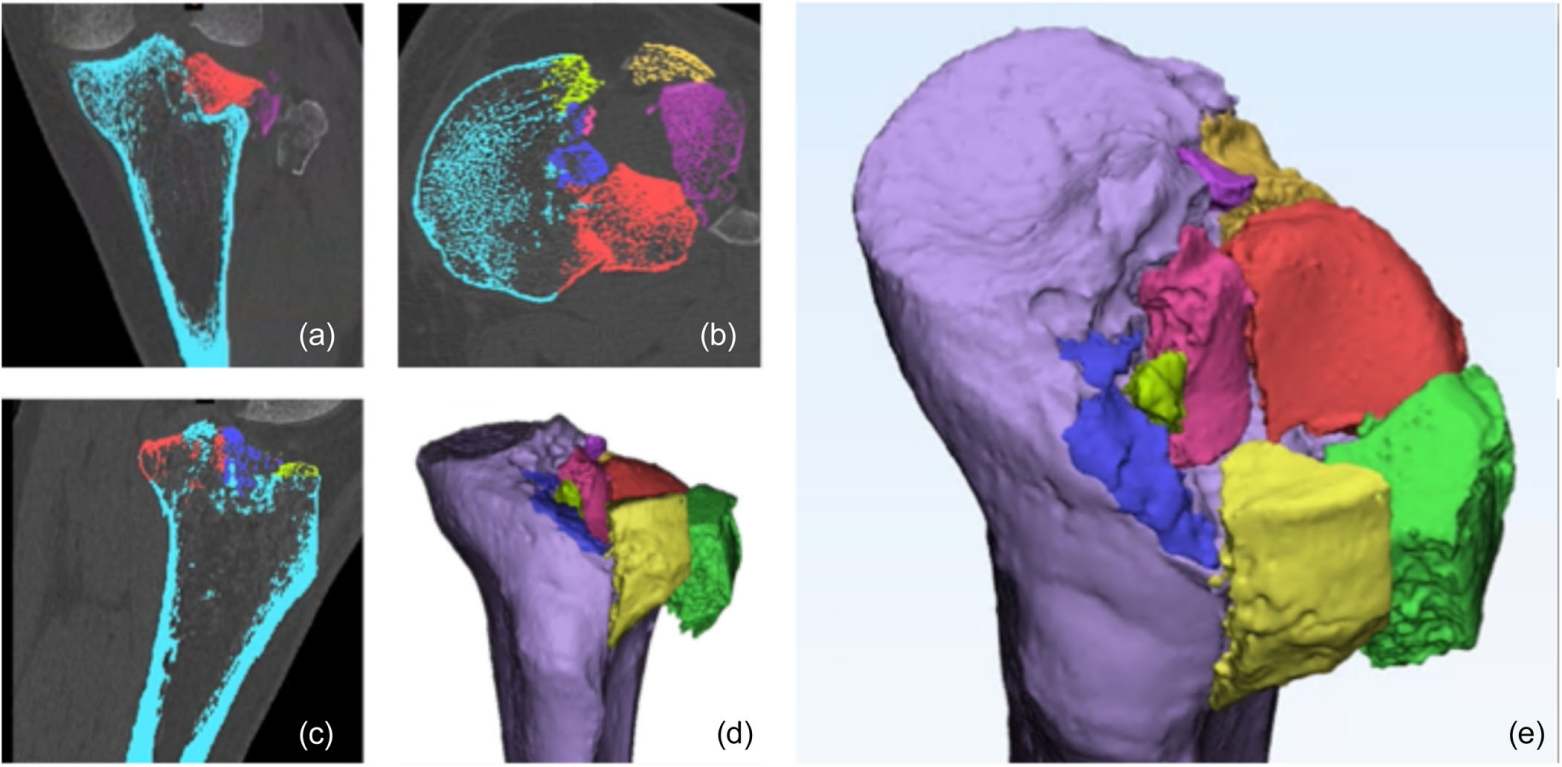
The segmentation process of a unicondylar tibial plateau fracture was conducted using a CT DICOM series (a–c) with the Materialise Interactive Medical Image Control system (Mimics Innovation Suite v24, Materialise, Leuven, Belgium). Following the initial segmentation process conducted with Materialise Mimics (d), the 3D model underwent post‐processing with manual local smoothing and surface fixing using 3‐Matic (e).
